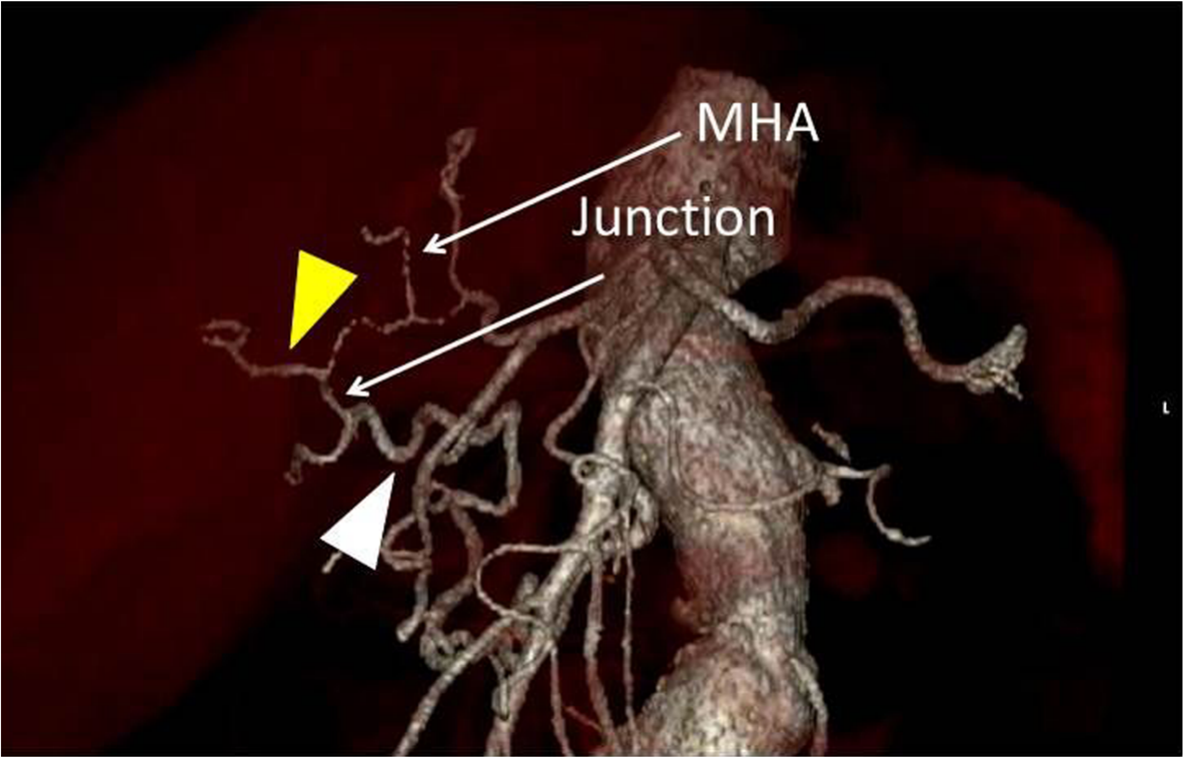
A 3D reconstruction of CT showed there were middle hepatic artery (labeled as “*MHA*”) and a junction (labeled as “junction”) between RHA-PB (*white arrow head*) and RHA-AB (*yellow arrow head*) at the hilar portion
